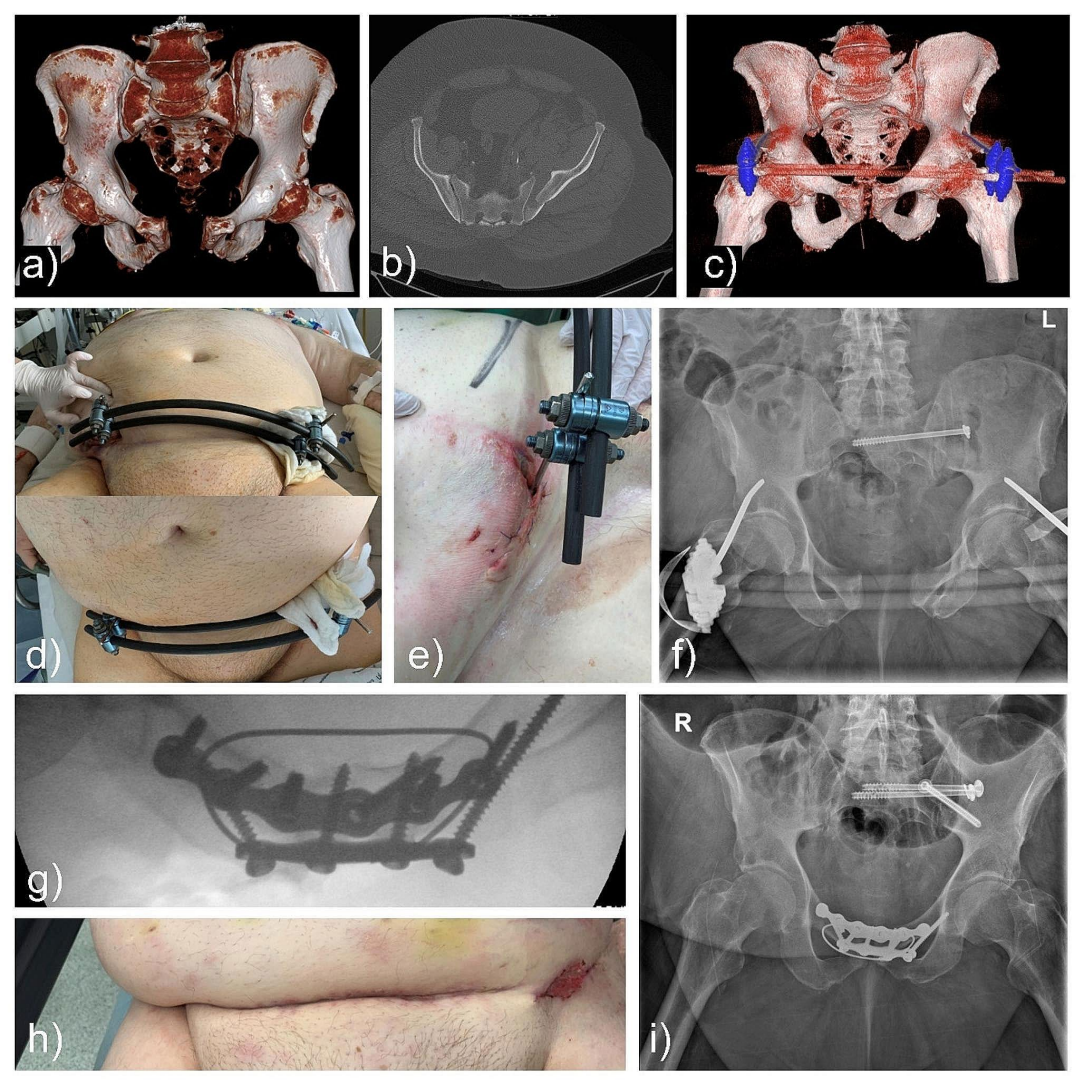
Case#5: a-c) An obese patient sustained a pelvic ring injury with symphyseal widening and a unilateral crescent fracture (AO: 61C1.1d). d-e) Common problems in these patients are pin-tract infections. f) Loss of reduction was observed in the x-ray image days later, before mobilization. g) Revision was done with double-plating and TOCF. Unfortunately, the patient had a surgical site infection. h) The infection was controlled after various wound debridements. i) The pelvic ring remained stable after mobilization
